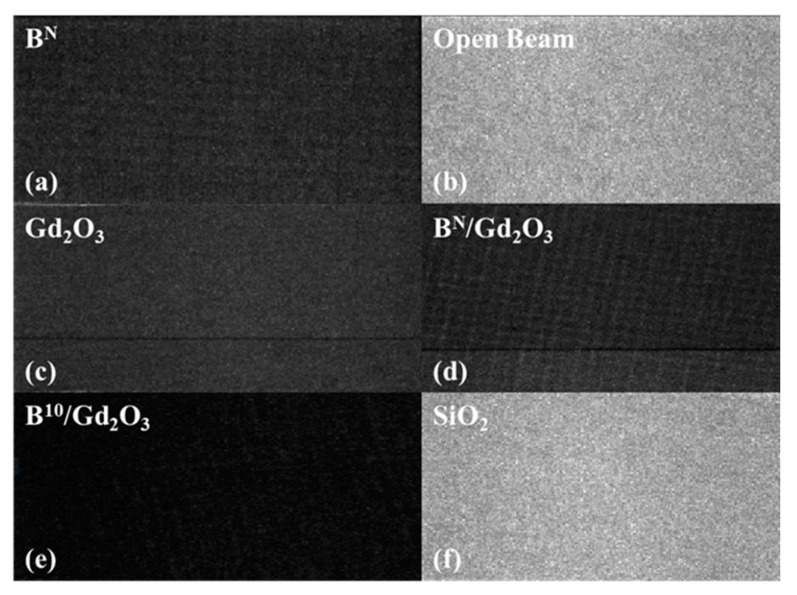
2D radiographs, summed over all neutron energies from 0.001 to 100 eV, of printed samples. For the formulations besides SiO_2_, the total filler content was 50 wt%. Lighter images correspond to more neutrons passing into the detector, while darker images correspond to a greater attenuation of incoming neutrons: (a) 50 wt% B^N^; (b) open beam (no sample); (c) 50 wt% Gd_2_O_3_; (d) 40/10 wt% B^N^/Gd_2_O_3_; (e) 40/10 wt% B^10^/Gd_2_O_3_; and (f) 10 wt% SiO_2_. All neutron-exposed pads were 3D printed using 500 um spacing.
